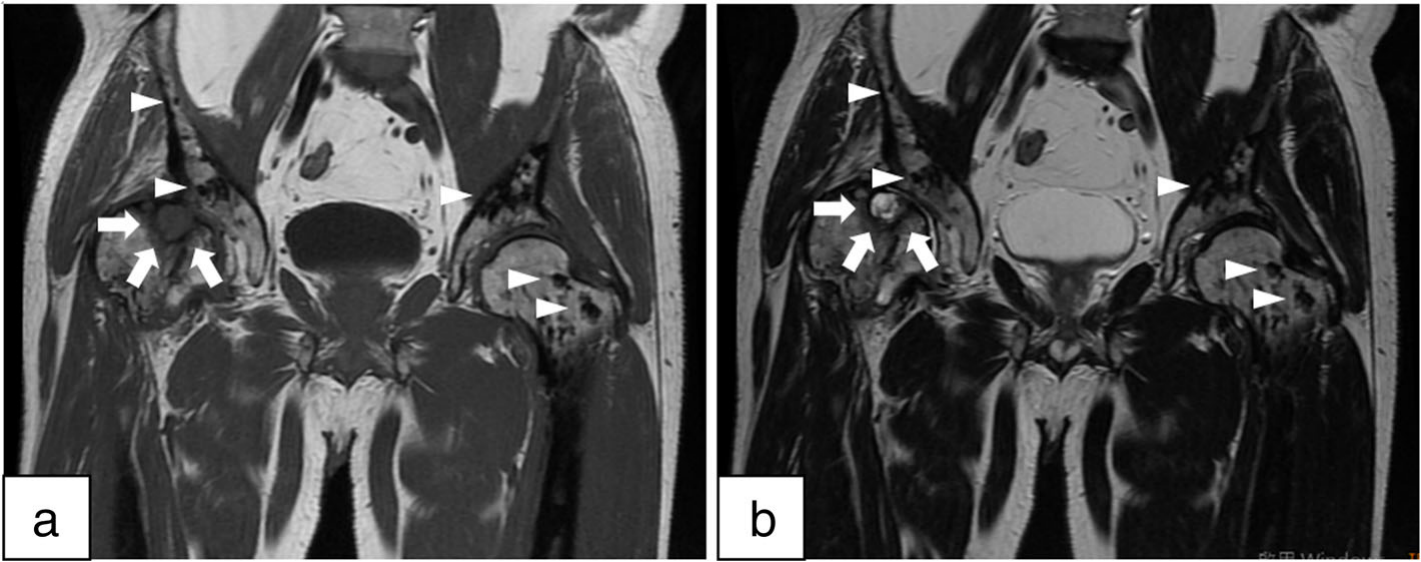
Coronal MRI of Both Hips. A subchondral cyst within the right femoral head, which was low intensity on T1-weighted (a) and high intensity with double line sign on T2-weighted images (b). (angled arrows). Multiple irregular lesions with low intensity in the pelvis and bilateral proximal femora on T1-weighted and T2-weighted images (a and b). (arrowheads)
